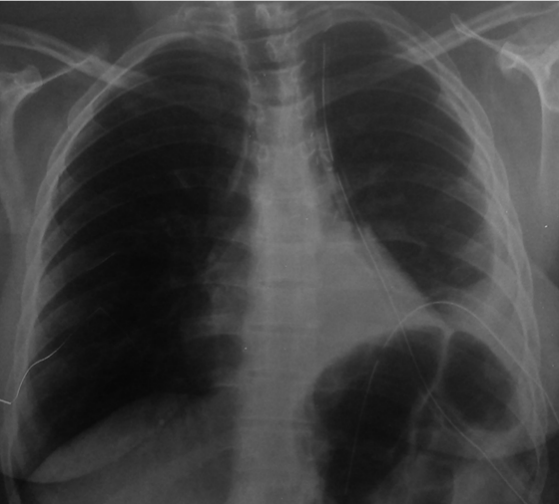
Radiographie thoracique post-opératoire
